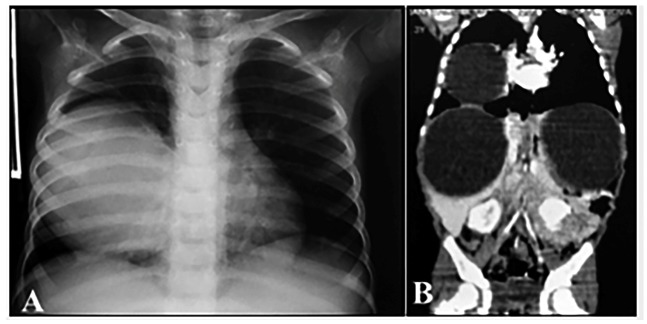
Anteroposterior chest radiograph (A) and coronal
